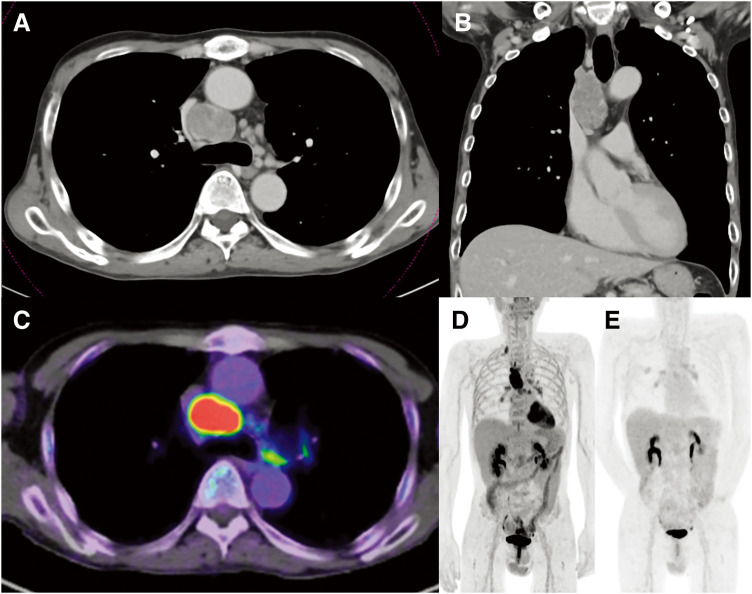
Preoperative findings. Chest CT revealed enlarged mediastinal LNs, measuring 72 × 37 × 31 mm in size. (A) Coronal view and (B) horizontal view. Preoperative PET-CT with FDG demonstrated tracer uptake (C) in the mediastinal LN and (D) faint accumulation in the bilateral hilar LN and the right subclavian LN. (E) Postoperative PET-CT performed 4 months after surgery showed no FDG accumulation suggestive of a primary tumor. FDG, 2-deoxy-2-(^18^F)-fluorodeoxyglucose; LN, lymph nodes
